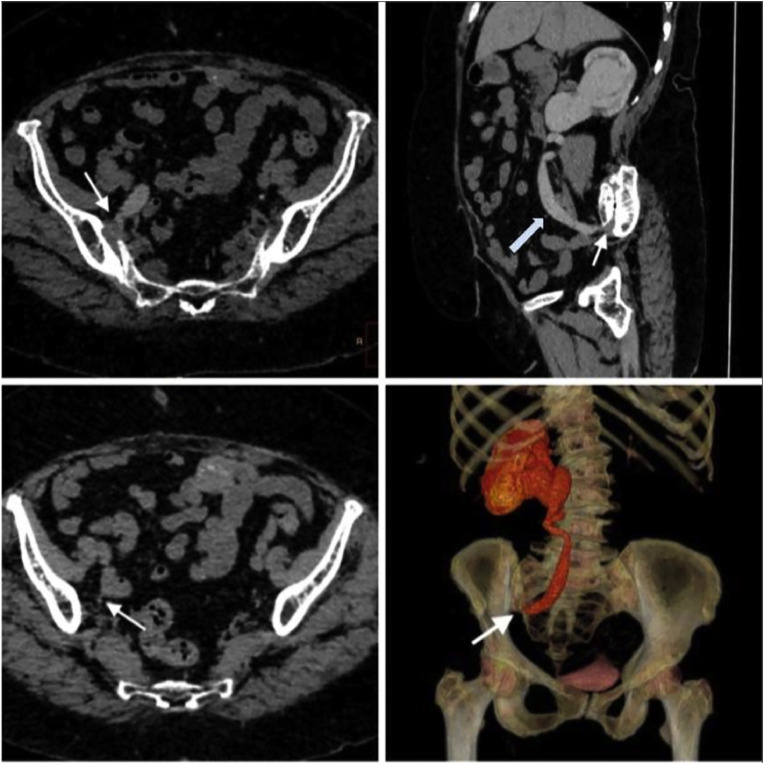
Nontraumatic right ureteral entrapment at the sacroiliac joint in a 77-year-old female. (A) Axial contrast-enhanced CT intravenous pyelography (CT IVP) image at the level of the sacroiliac joints. There is moderate dilatation of the right ureter (white arrow) terminating abruptly at the anterior aspect of the right sacroiliac joint. (B) Sagittal reformatted CT image clearly visualizing the focal narrowing and entrapment of the right ureter within the irregular margins of the degenerative sacroiliac joint (white arrow) and dilated proximal ureter (long arrow) obtained on 12 hours scan. (C) Axial CT image through the lower pelvis, distal to the site of entrapment. The distal right ureter appears narrow and nondilated (white arrow), confirming the level of obstruction. (D) Three-dimensional (3D) volume-rendered CT reconstruction of the image obtained after 12 hours was done using Philips intellispace portal providing a global view. The dilated right ureter is seen descending and terminating at the level of the right sacroiliac joint (white arrow). Note the associated ureteral kinking superiorly.
